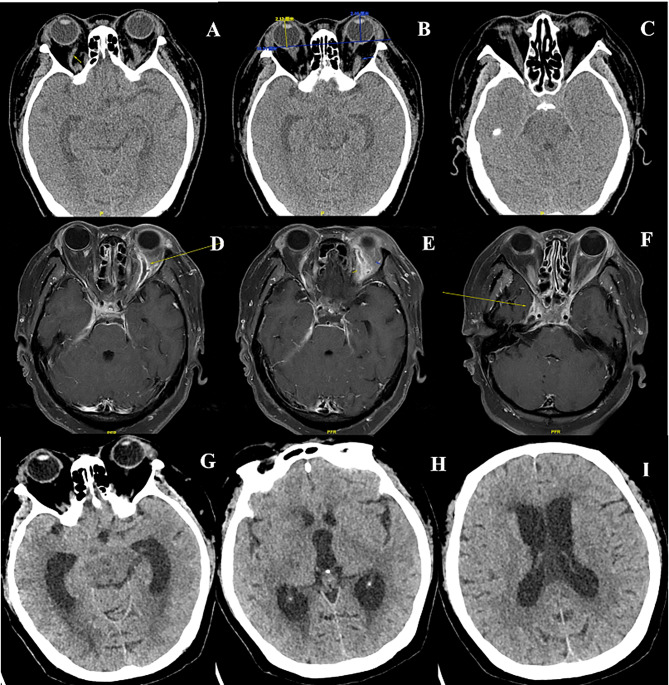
Serial neuroimaging findings. (A–C) orbital CT (August 23): bilateral proptosis and periorbital edema, dilation of the superior ophthalmic veins, and inflammatory opacification of the paranasal sinuses. (D–F) orbital MRI (August 23): confirms bilateral proptosis and dilated superior ophthalmic veins, suggestive of thrombophlebitis. (G–I) cranial CT (September 11): marked enlargement of the third and bilateral lateral ventricles, consistent with hydrocephalus; no evidence of hemorrhage
